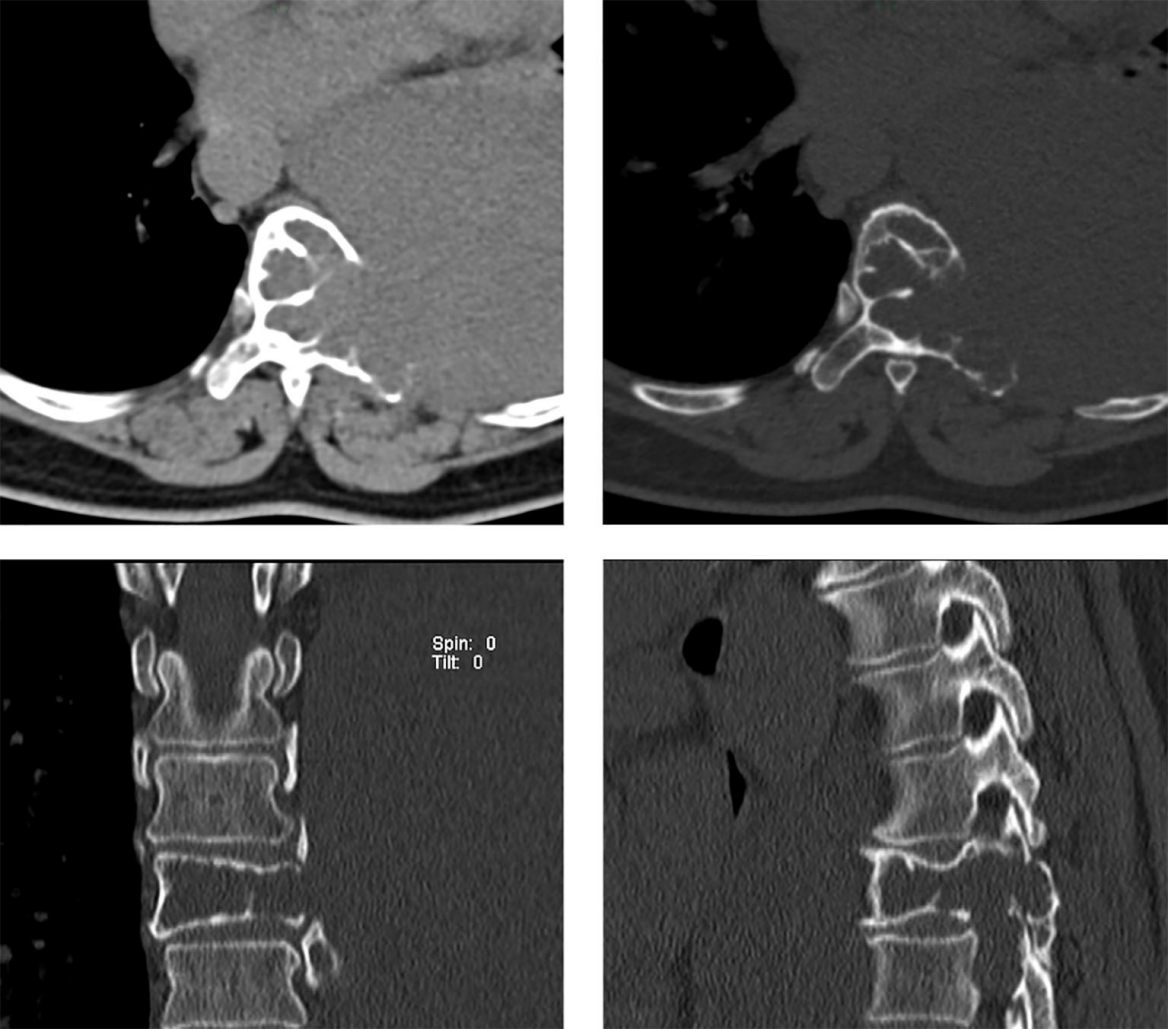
CT manifestations of Case 2 (T8 vertebra). Axial CT scan reveals bone destruction in the T8 vertebrae and the left costotransverse joint, and irregular soft tissue density shadows were observed in the T8 vertebra, which protruded into the thoracic cavity on the left side.
